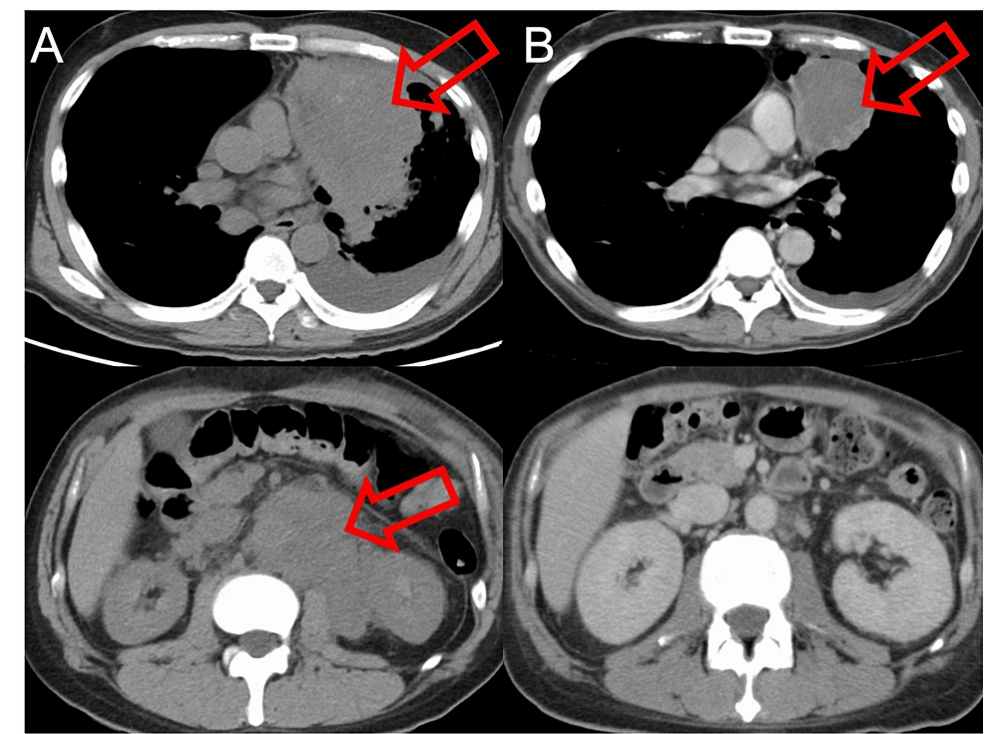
Case 2 (A) Before treatment, chest CT showed a 10-cm tumor in the left upper lobe of the lung (indicated by red arrow) and a left pleural effusion (upper), and abdominal CT showed a large tumor clustered together with lymph nodes (indicated by red arrow) (lower); (B) Two months after starting ICIs in combination with cytotoxic chemotherapy, chest CT showed shrinkage of the left upper lobe tumor (indicated by red arrow) and reduction of left pleural effusion (upper), and abdominal CT showed marked reduction of the abdominal tumor (lower). ICI: Immune checkpoint inhibitors
